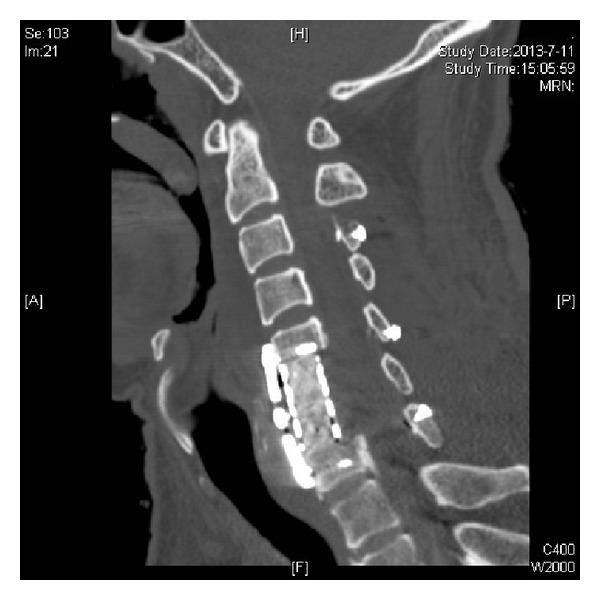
Postoperative CT-scan sagittal reconstruction. A C3–C7 laminoplasty and C6 corpectomy with reconstruction using a titanium mesh cage and plate has been done. The spinal canal is patent.
